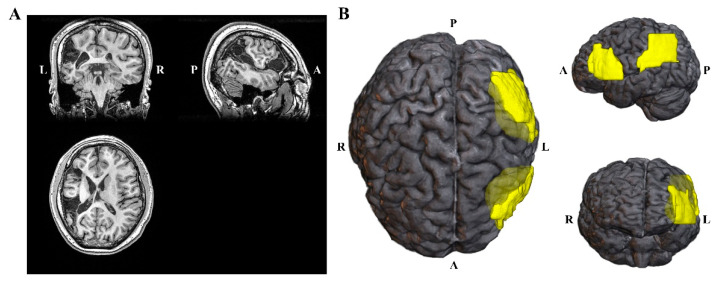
Three-dimensional (3D) MRI image and the locus of brain lesions. (A) T1-weighted MR brain images of participant. (B) Lesion map of participant, yellow areas represent brain lesions.
